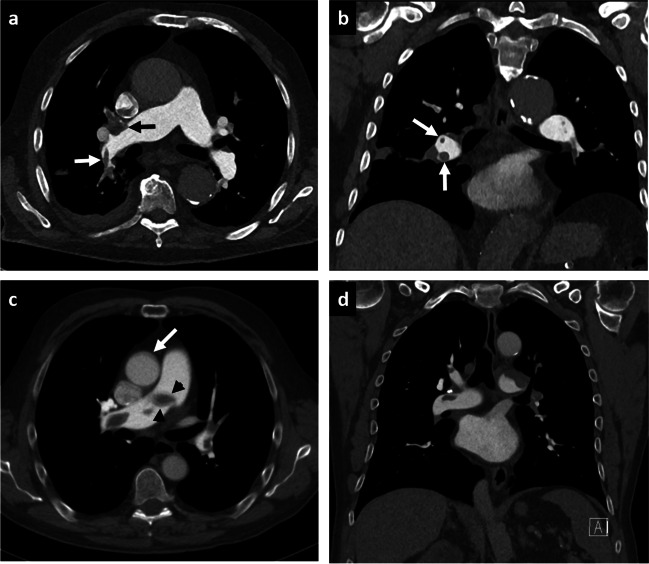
PCD-CT (a, b) and EID-CT (c, d) demonstrating bilateral pulmonary embolism. Dual-energy EID-CT exhibits significant pulsation artifacts (c; arrow) and blurring of thrombus material (c; arrowheads) compared with high-pitch 60-keV PCD-CT. Despite application of a low-dose protocol, PCD-CT delivers excellent intraluminal contrast, paired with high image sharpness and delineation of the thrombus material (a, b; arrows). *PCD-CT*, photon-counting detector CT; *EID-CT*, energy-integrating detector CT
